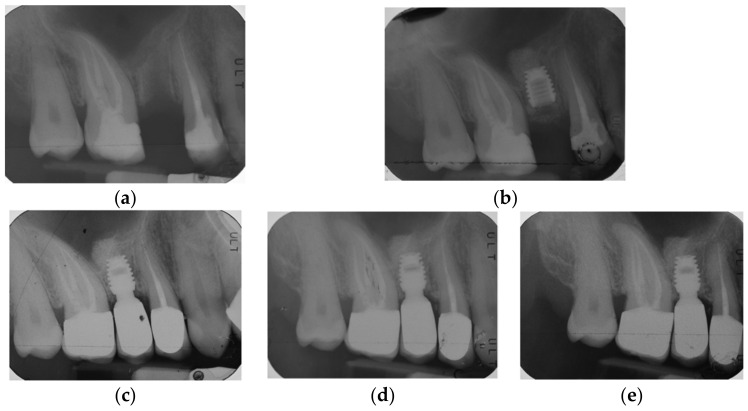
Single implant placed in 1.5 site (4.5 × 6 mm): (a) pre-operative radiograph before implant placement; (b) radiograph obtained at time of placement; see augmented sinus floor (sinus lift = 3.33 mm); (c) radiograph obtained at time of loading; (d) radiograph obtained at 3-year follow-up; (e) Radiograph obtained at 5-year follow-up. See minimal graft resorption after 3 years and stable bone levels with minimal changes between 3-year (RCBH = 6.5 mm; sinus lift = 3.1 mm) and 5-year follow-up (RCBH = 6.2 mm; sinus lift = 2.8 mm).
